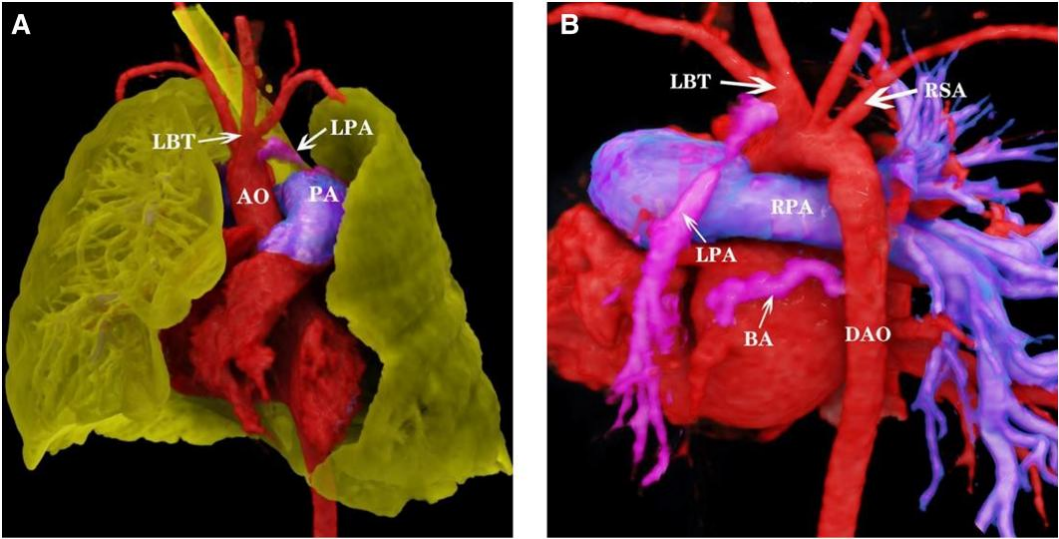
A 1-month-old boy’s left pulmonary artery heterotopia originated from computed tomography angiography of the left brachiocephalic trunk. (*A*, *B*) Cinematic rendering images showed that the left pulmonary artery heterotopic originated from the left brachiocephalic trunk. AO, ascending aorta; BA, bronchial artery; DAO, descending aorta; LBT, left brachiocephalic trunk; LPA, left pulmonary artery; PA, pulmonary artery; RPA, right pulmonary artery; RSA, right subclavian artery.
